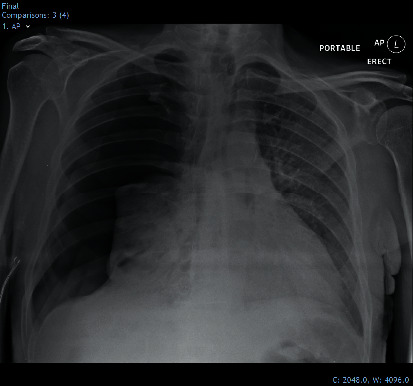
Portable chest radiograph following acute deterioration, demonstrating a right-sided tension pneumothorax.
